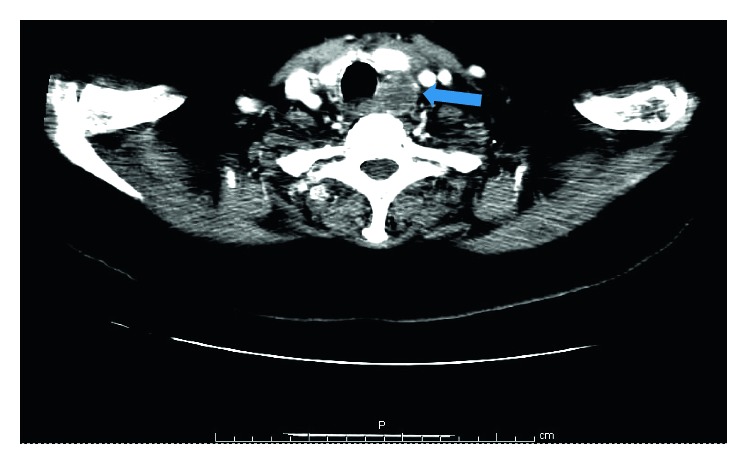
Neck CT showing a left parapharygeal/paralaryngeal nonenhancing mass (blue arrow) with 2.3 cm of maximum diameter.
